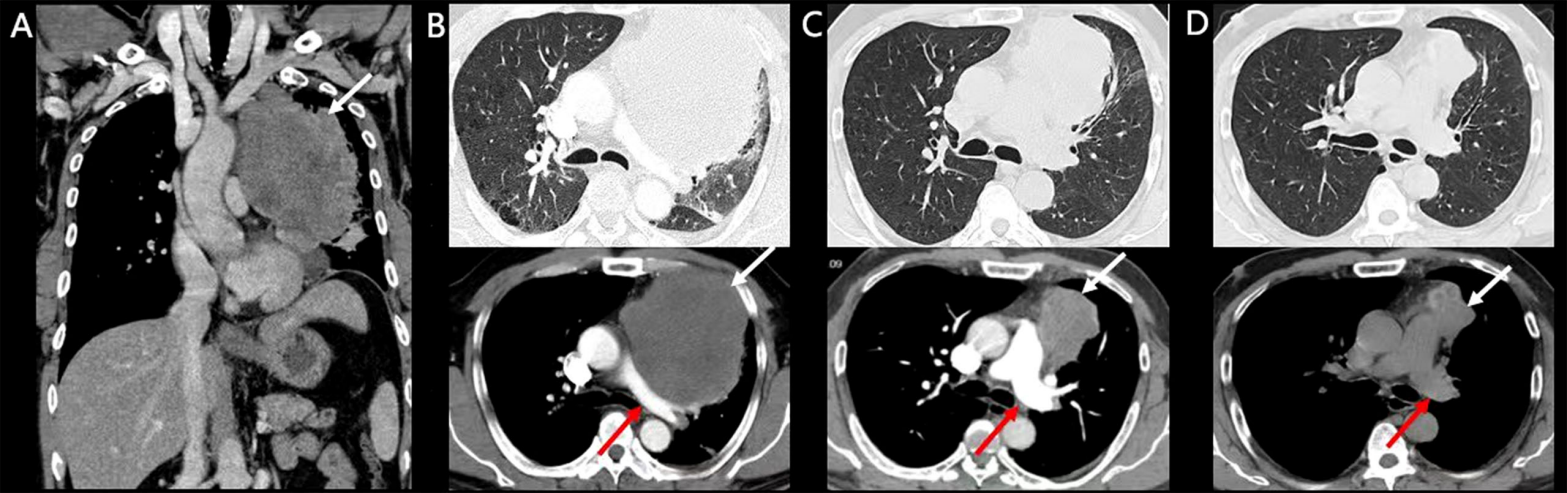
Enhanced CT and follow-up CT images. White arrows indicate tumors and red arrows indicate the pulmonary artery. (A, B) Pretreatment CT scan of the patient. (C) CT scan after two doses of Tislelizumab. (D) CT scan after six doses of Tislelizumab.
